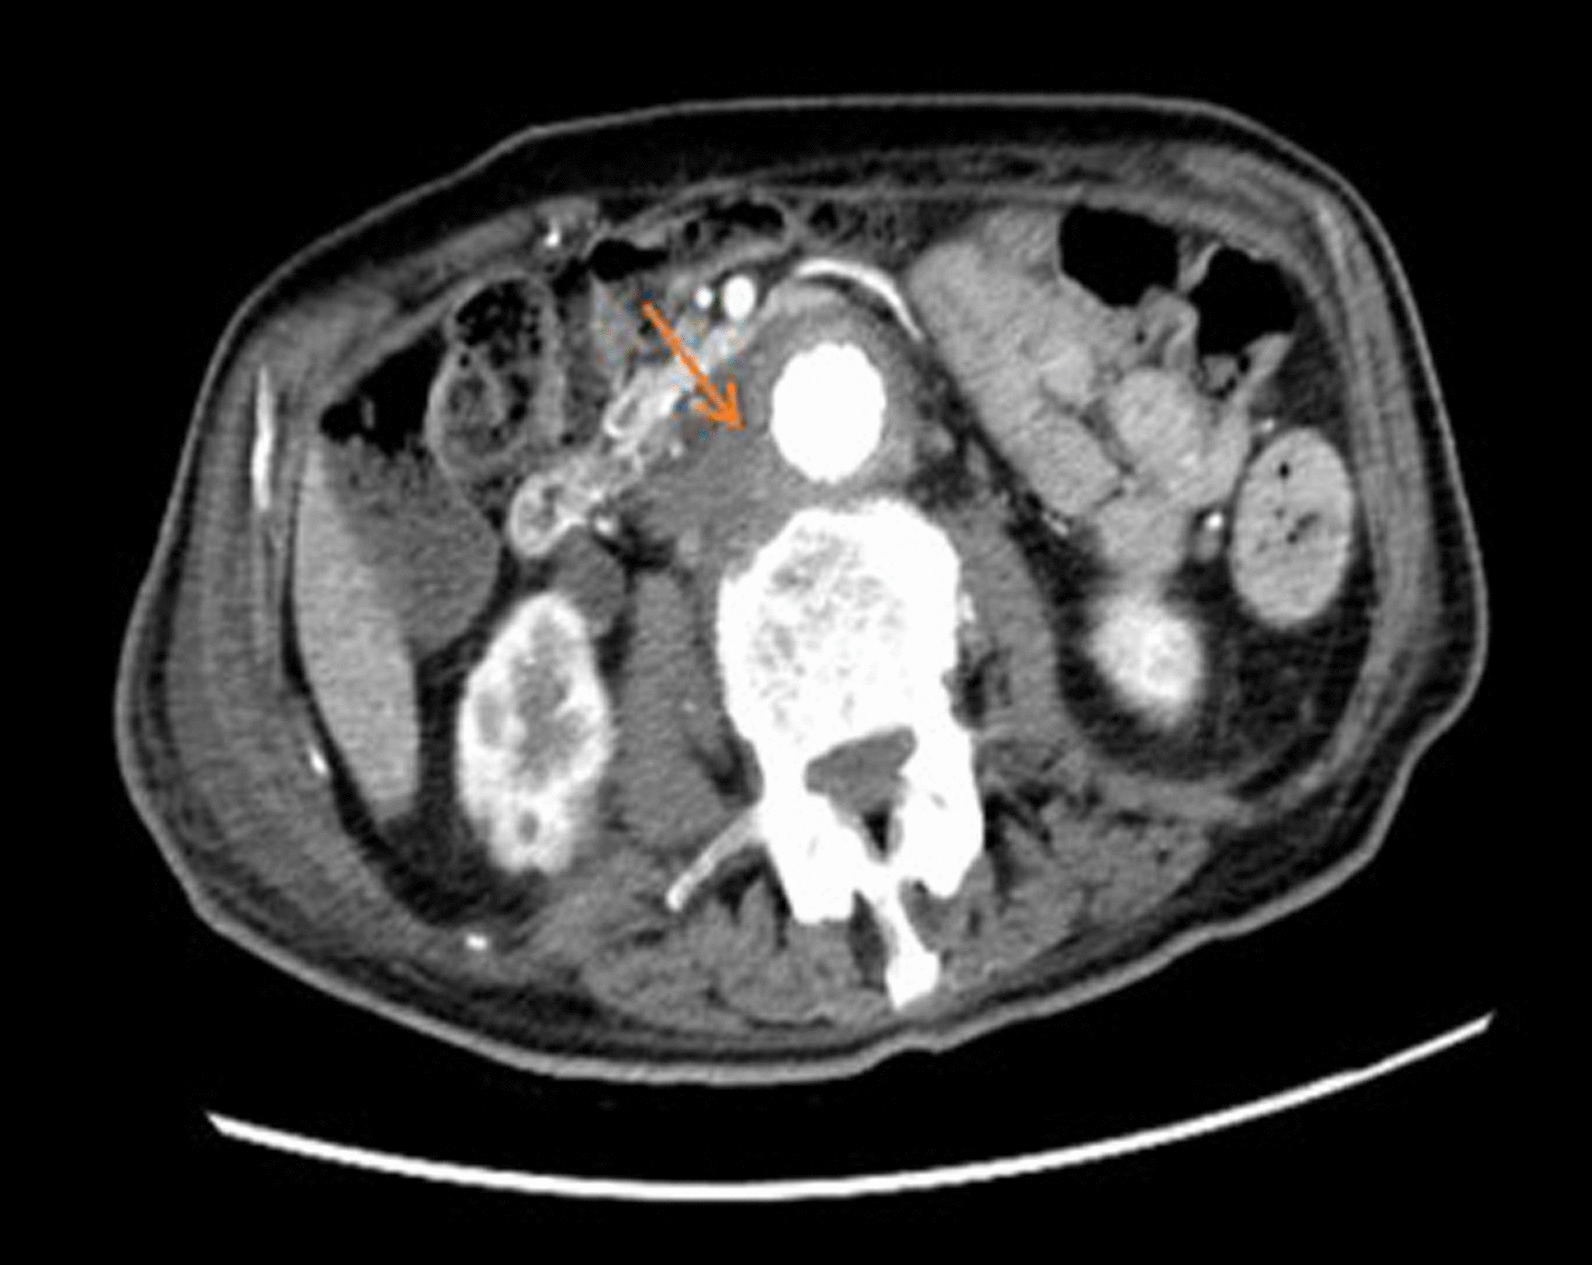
Computed tomography (CT) showed equi- or low-density soft tissue collection around the aorta and an unclear boundary between the aorta and bowel
